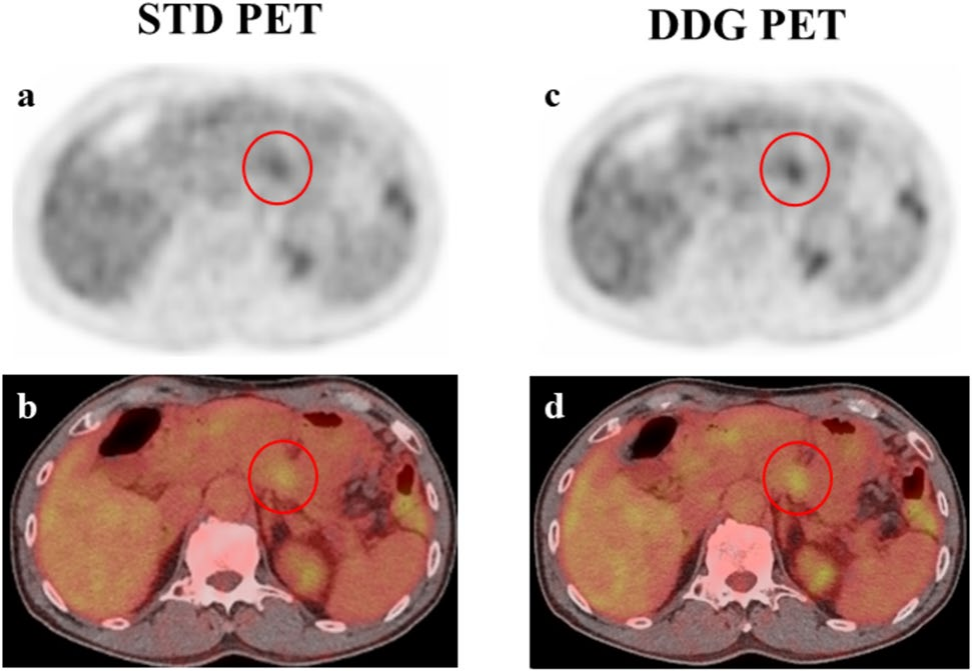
Pancreatic cancer. The pancreatic cancer lesion was visualized with less blurring and more clearly in DDG than in STD FDG PET. The scores of lesion blurring and conspicuity were 0 and 0 in STD and 1 and 1 in DDG PET images, respectively. *STD*: standard free-breathing, *DDG*: data-driven respiratory gating. a, c: Transaxial FDG PET image, b, d: fused FDG PET/CT image
